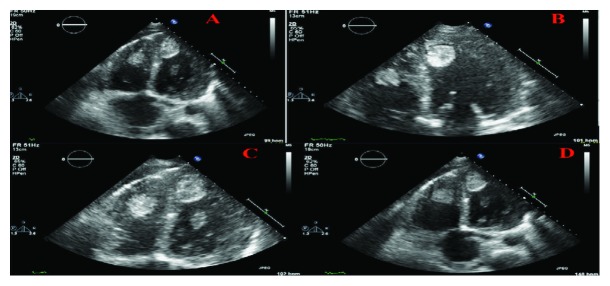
Transthoracic echocardiography, apical 4-chamber views, and multiple pedunculated 2-3 cm echodensities in the right and left ventricles consistent with intracardiac thrombi (a–d): moderate left ventricular hypertrophy (a), borderline LV dilatation (b), right ventricle appears moderately dilated (c), and intra-atrial septal deviated to the left (d). There is a small pericardial effusion.
